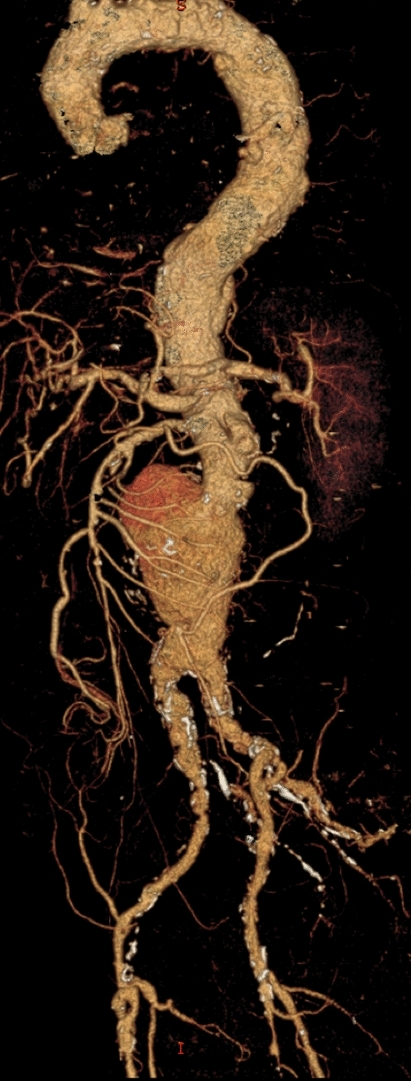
3-D CT aortogram reconstruction showed the lateral bulge of the abdominal aortic aneurysm and severely diseased and ectatic aorta
